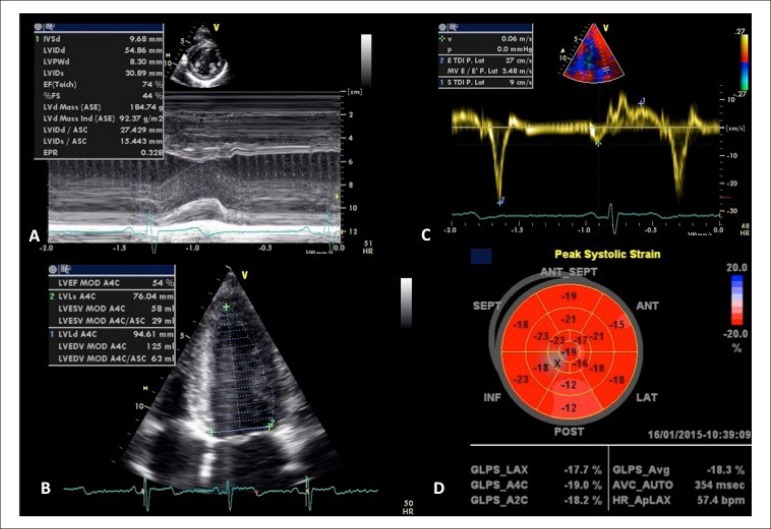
Transthoracic echocardiogram at rest without evidence of significant
